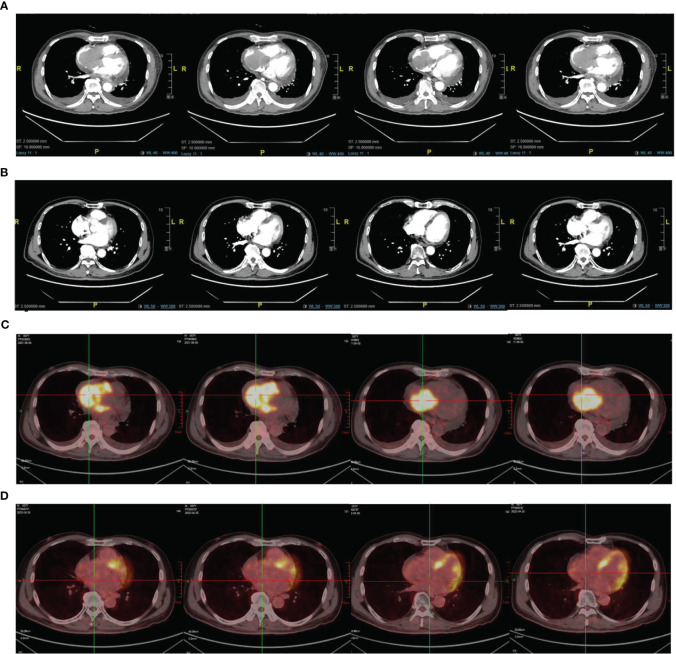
(A) Image of chest CT scan on August 3, 2021. (C) PET/CT scan on August 6, 2021. (B) Image of chest CT scan on April 3, 2022 (D) PET/CT scan on April 6, 2022.
